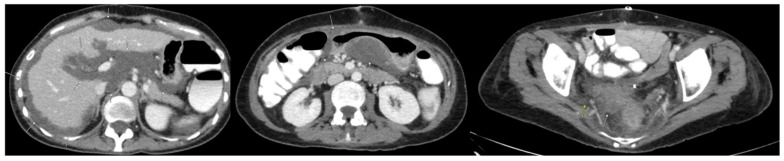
CT scan of the abdomen/pelvis conducted after first CRS/HIPEC. Arrows indicate residual mucinous peritoneal metastases and demonstrate interval reduction in tumor burden.
